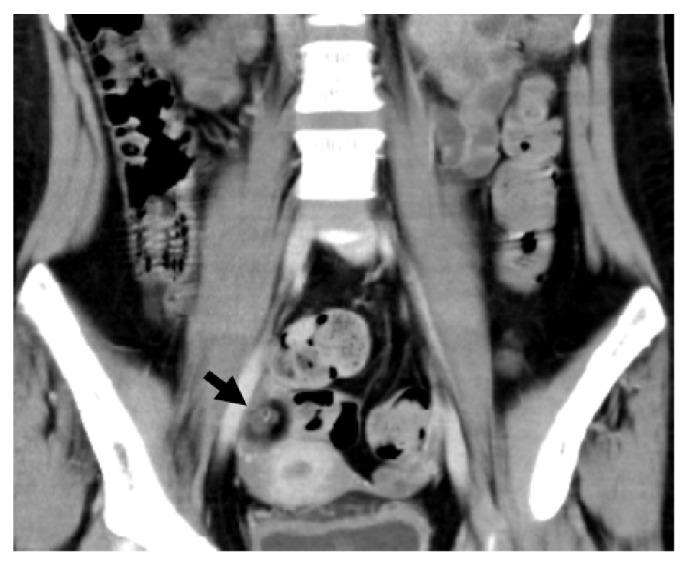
Abdominal CT after 8 months of admission demonstrating a small ovarian calcified cystic mass on the right side (arrow).
